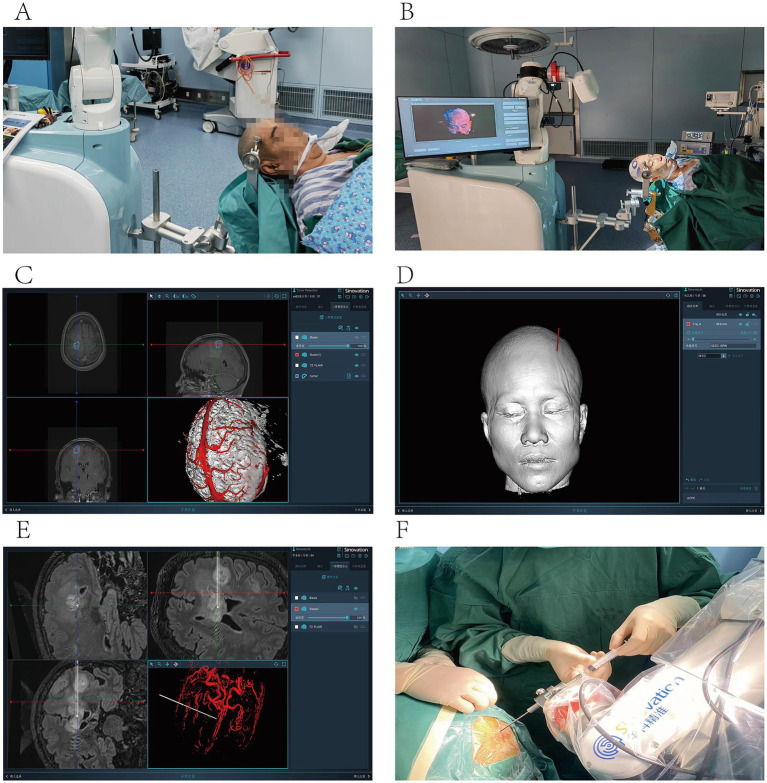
(A) After the patient’s head protection part is fixed by the Head immobilization device, push the robot to the front end of the mechanical arm base, which is 50–55 cm away from the midline of the patient’s external auditory canal. (B) The locator collects head and facial data from different angles. (C) The Huake Precision robot biopsy software integrates preoperative cranial MRI and preoperative localized cranial CT images. (D) The reconstruction of head and facial images was successful. (E) Calculate the puncture target and the approach to the skull, avoid the sulci and important blood vessels, and take the preoperative target as the objective. (F) The robot located the puncture target, successfully inserted a 2.5 mm biopsy needle, and slowly extracted the tissue.
